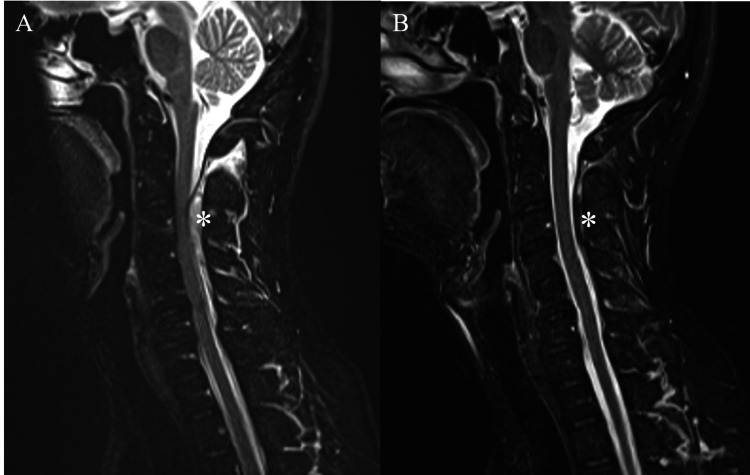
Hematoma in the case of conservative treatment (A) and (B) reveal the change of hematoma in a patient who has received conservative treatment. (A) A typical magnetic resonance imaging appearance of C2-C6 spontaneous spinal epidural hematoma (*) on sagittal T2-weighted imaging on admission. (B) The hematoma (*) decreased but did not resolve completely after five days of conservative treatment.
